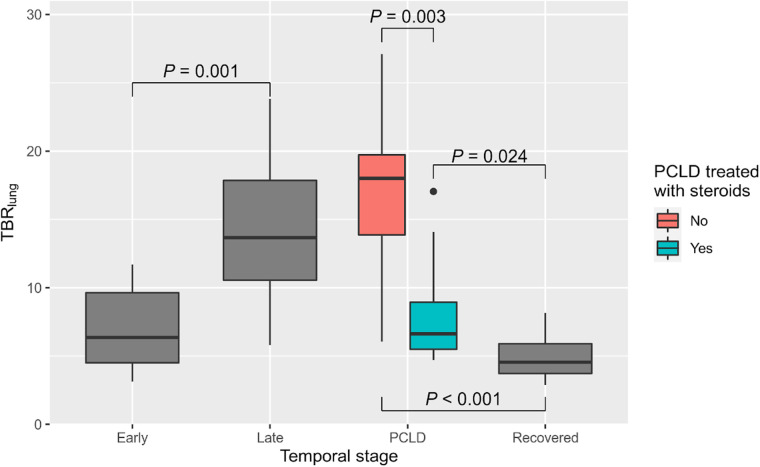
^18^F-FDG uptake (TBR_lung_) by temporal stage.
